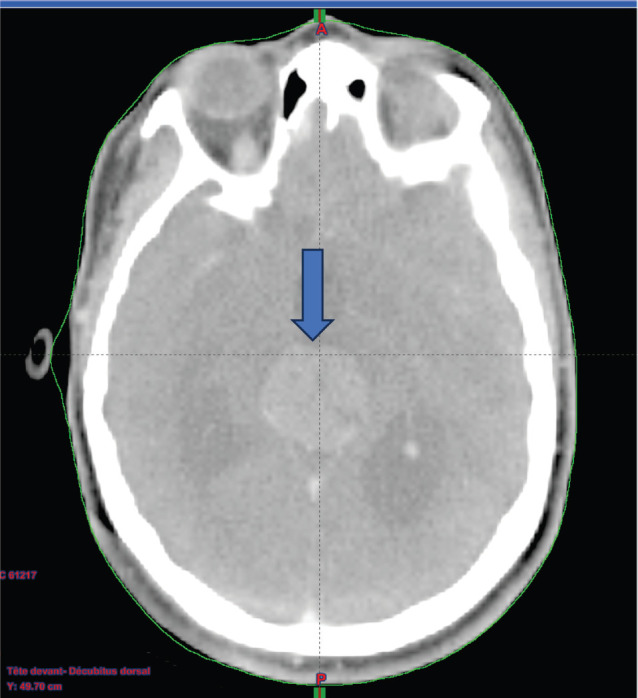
A contrast-enhanced brain CT scan reveals findings suggestive of a tumour in the pineal region.
